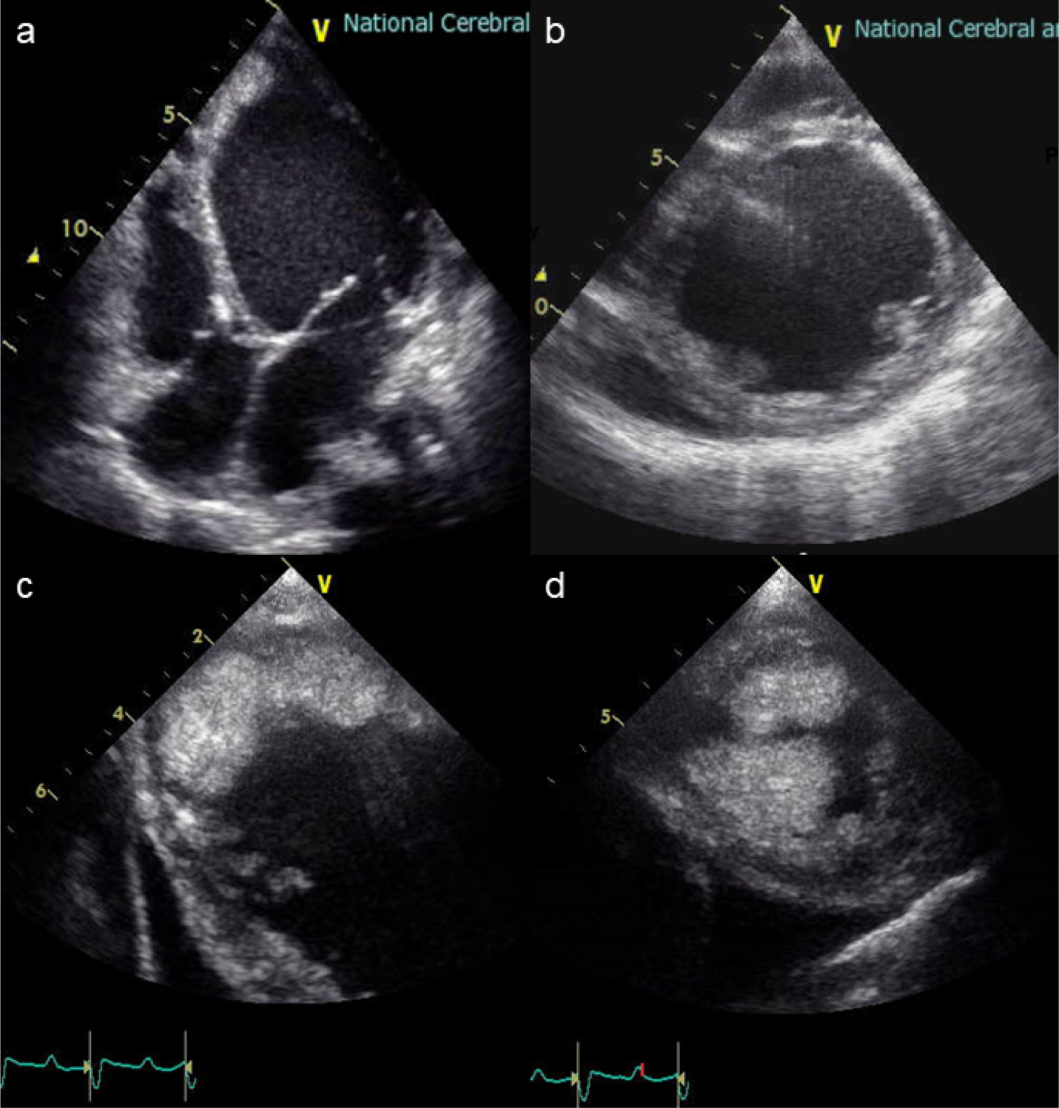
Transthoracic echocardiography findings before paracorporeal left ventricular assist device implantation. (a,b): Echocardiography showed remarkable dilation of the left ventricle. The right ventricular volume was not excessive. (c, d): Echocardiography showed a huge thrombus on the apical side of the left ventricle
